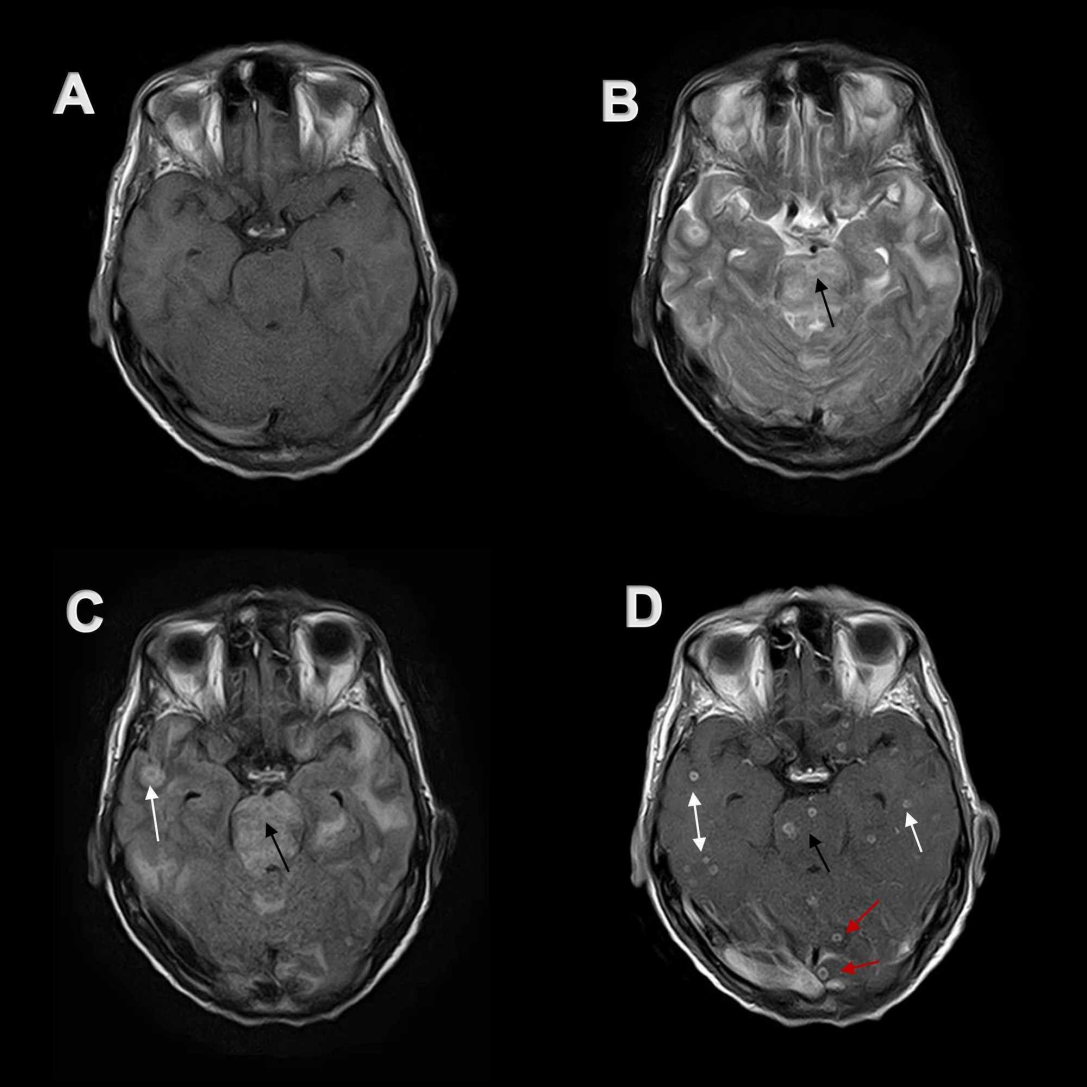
Axial MR imaging of the brain at the level of pons Numerous lesions are present in bilateral temporal lobes (white arrows) and occipital lobes (red arrows) along with two lesions in the pons (black arrows). (A) Lesions are isointense on axial T1-weighted MR image. (B and C) Same lesions appear hypo-intense on axial T2-weighted MR image and axial FLAIR MR image. (D) These lesions show avid ring contrast enhancement on post-contrast axial T1-weighted MR image. MR, magnetic resonance. FLAIR, fluid-attenuated inversion recovery
